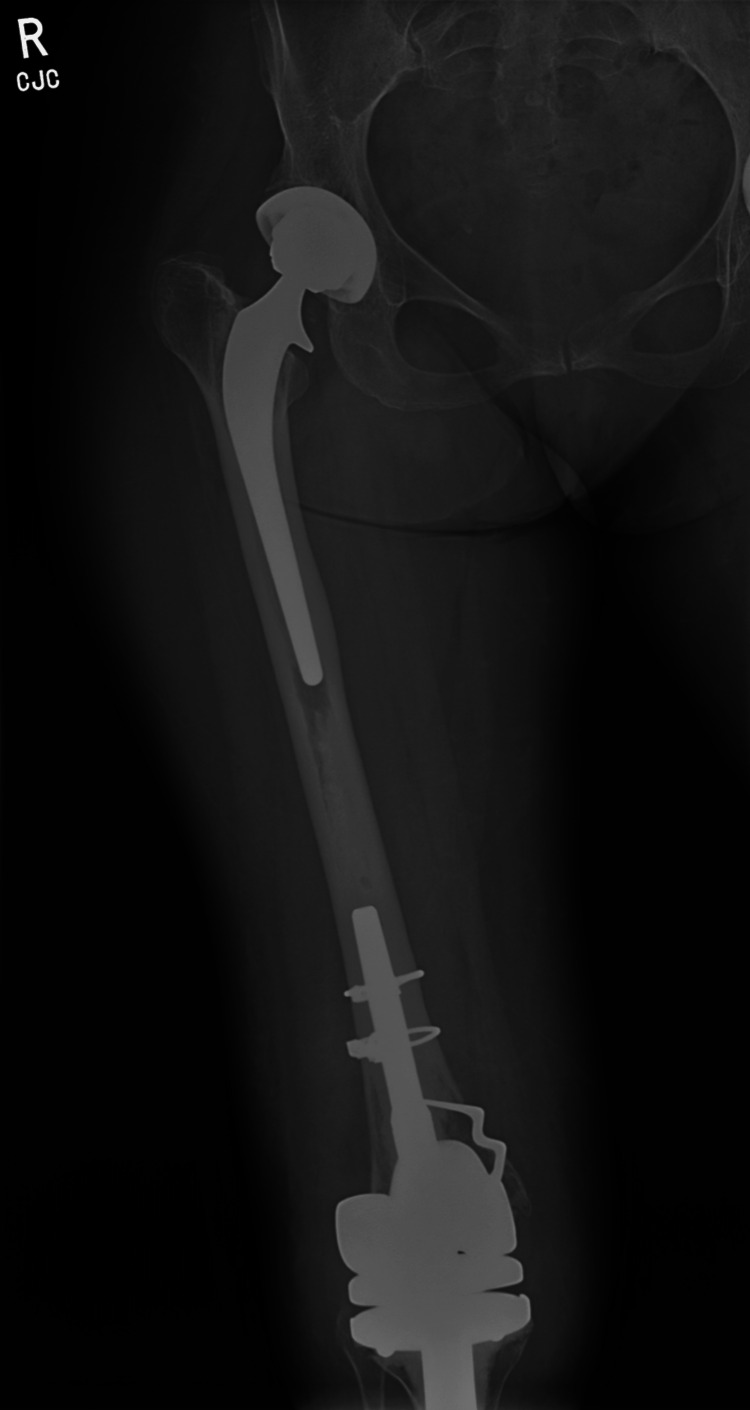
Preoperative anteroposterior X-rays of the patient showing the total hip replacement and the infected total knee replacement of her right leg
